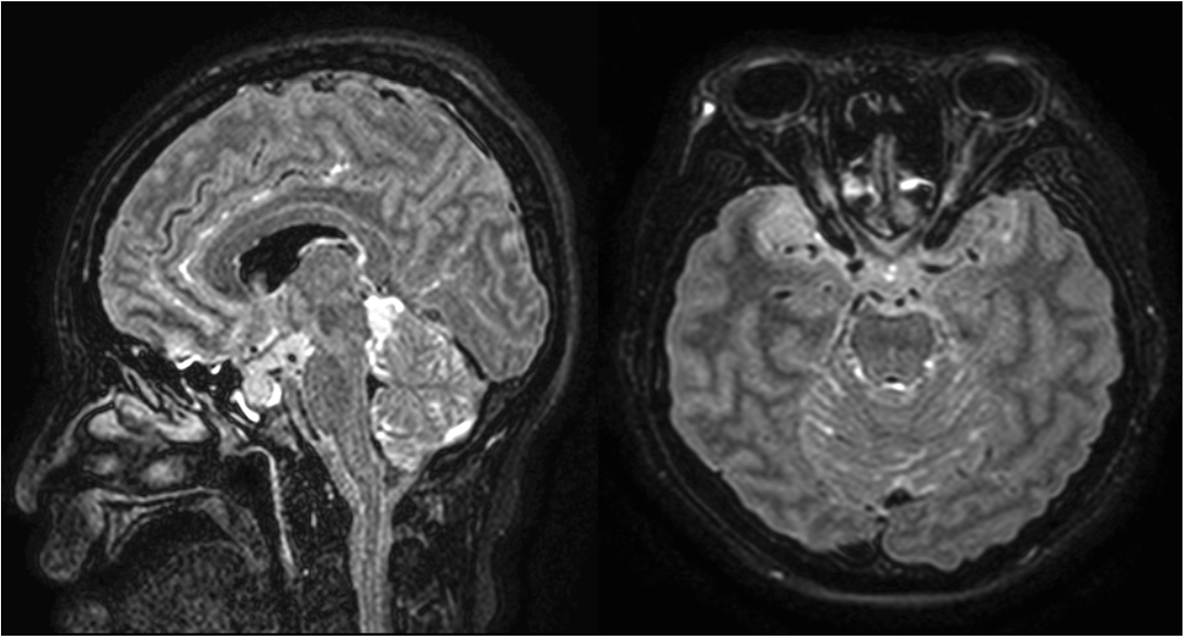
Brain MRI showed extension of glioblastoma of spinal cord to the brainstem and another lesion in the right temporal lobe
